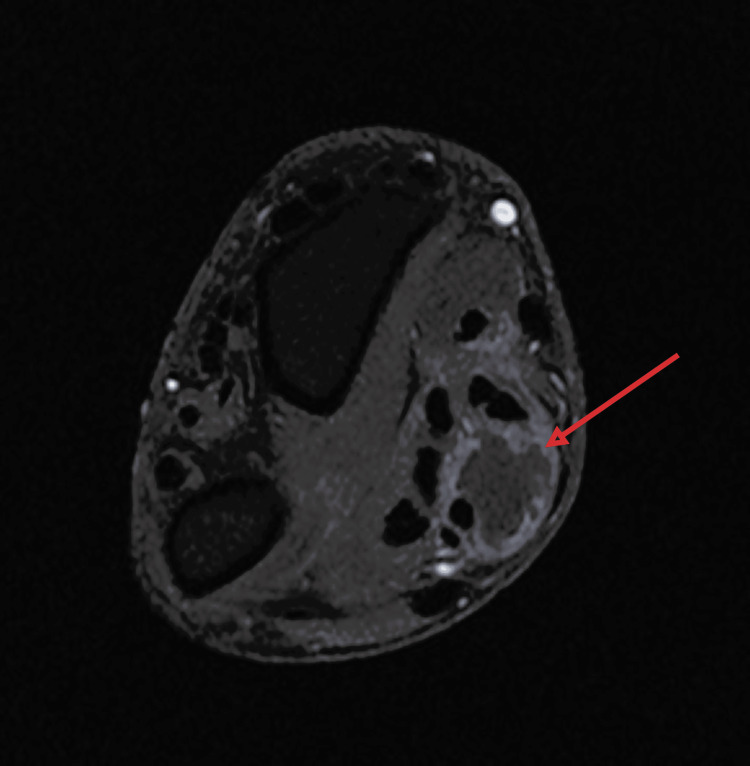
T1 fat-saturated axial MRI of the wrist The image is showing a well-defined fluid collection along the flexor tendon sheaths, suggestive of tenosynovitis.
